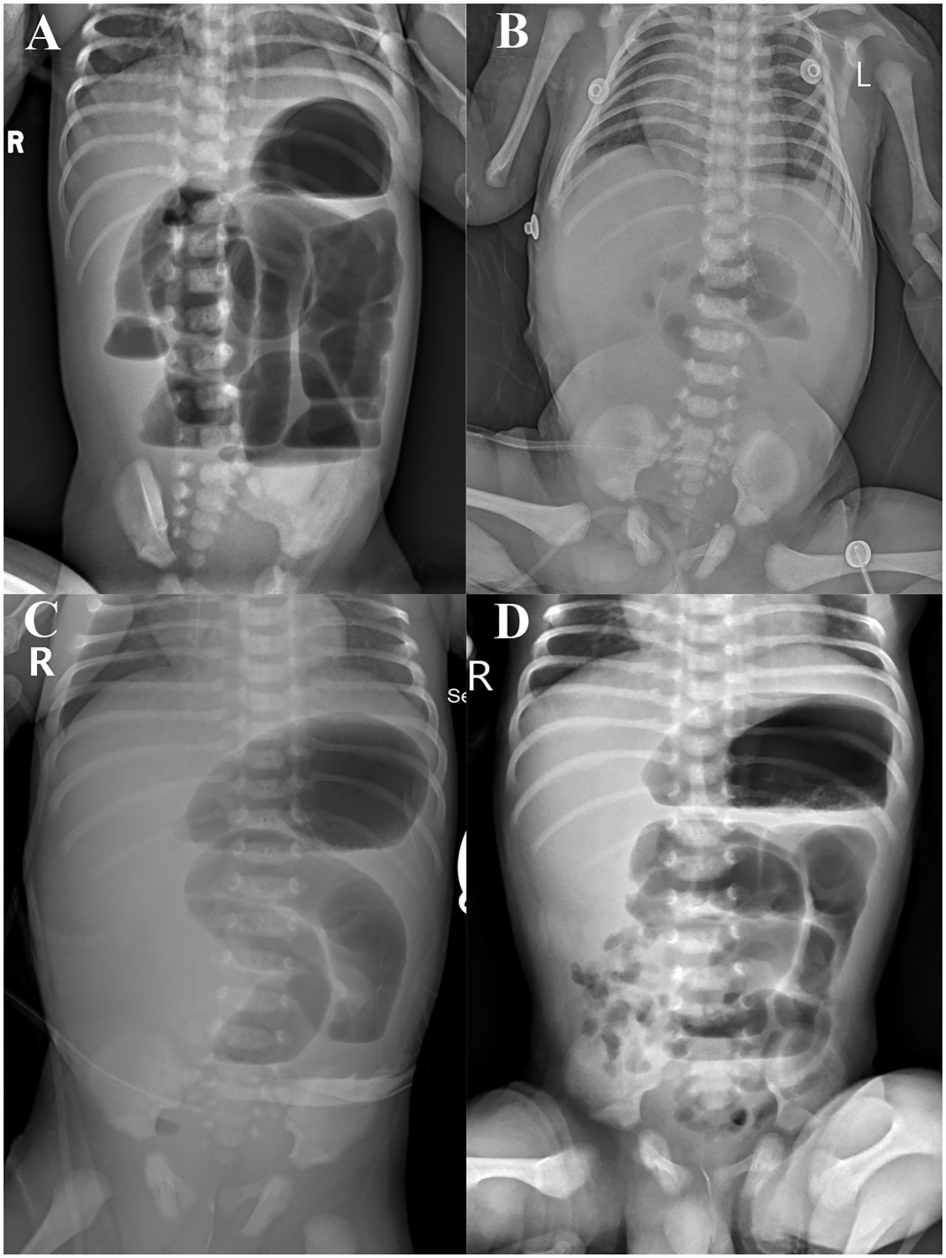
Abdominal x-rays before (A) and after (B–D) the first surgery.
